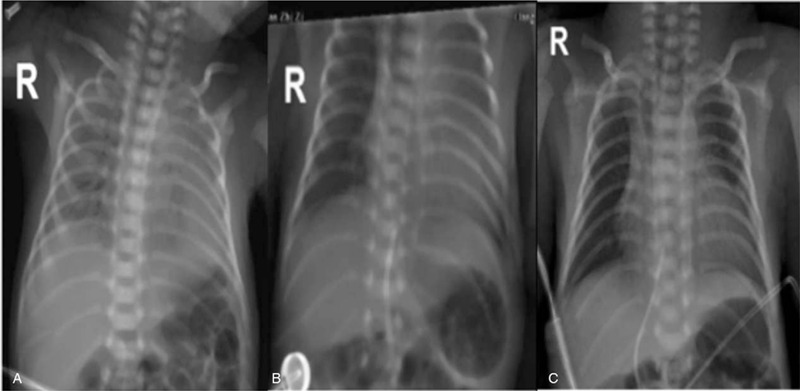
A: Initial chest radiograph showing white lung before surfactant. B: Chest radiograph two hours after surfactant showed a good improvement in lung translucency. C: Chest radiograph showed significant improvement four hours after nHFOV.
